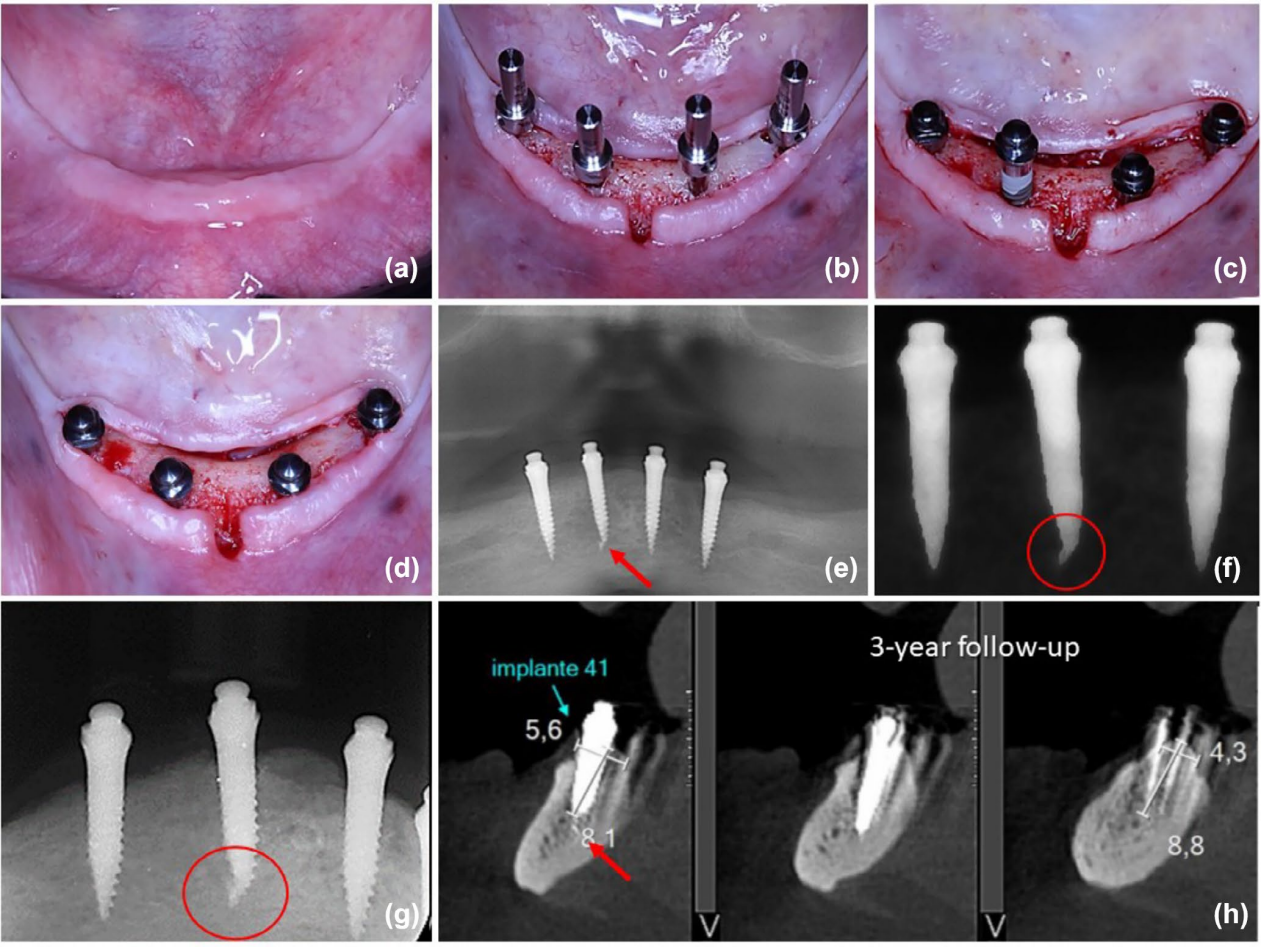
(a) Presurgical view of the edentulous mandible ridge; (b) Transoperative view of flapped surgery; (c) Insertion of mini implant in the central right position; (d) Intraoral aspect of the placed mini implants; (e) Panoramic radiograph at 6‐week follow‐up evidencing a radiopaque image suggestive of mini implant tip fracture; (f) 3D reconstruction of CBCT showing the position of the fractured fragment using a boneless filter; (g) Periapical radiograph at 2‐year follow‐up of the central right implant evidencing the fractured implant tip; (h) CBCT image scans at the 3‐year follow‐up, showing the fractured fragment located in a dense cortical bone and revealing satisfactory osseointegration.
